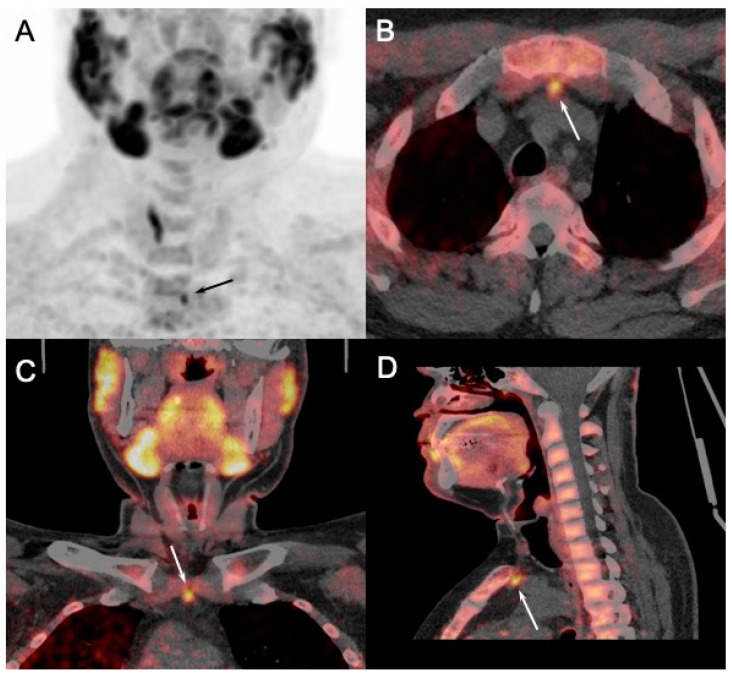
[^18^F]FCH PET/CT ((A): anterior MIP; (B–D): axial fusion images) performed in 40-y-old MEN1 patient with recurrent primary hyperparathyroidism (PTH: 290 ng/L; calcemia: 2.66 mmol/L) and previous history of left thyroidectomy, superior left, and inferior bilateral parathyroidectomy. Cervical US failed to detect pathological glands. PET/CT showed pathologic [^18^F]FCH uptake in 8mm retrosternal soft tissue nodule, suggesting ectopic parathyroid tumor afterwards confirmed by pathology after surgical excision.
